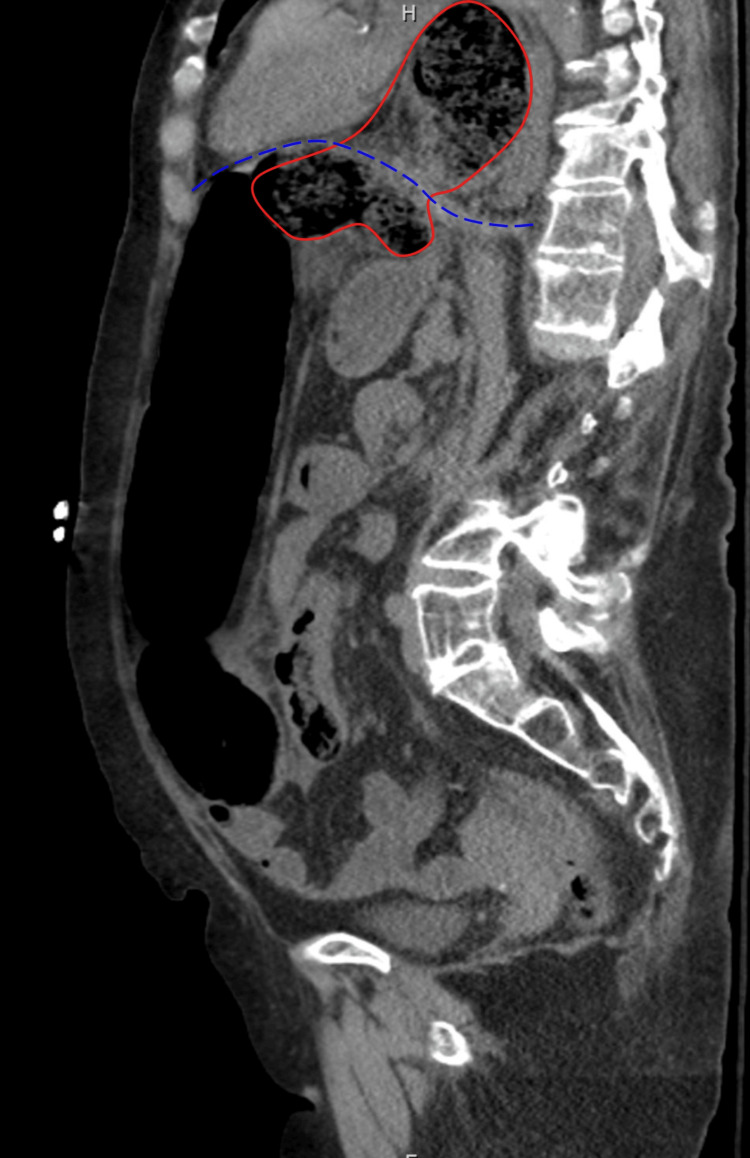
A sagittal view of the CT abdomen and pelvis shows a dilated bowel anteriorly (left) and a necrotizing bowel herniating into the thoracic cavity. The incarcerated apex of the sigmoid volvulus is shown, delineated in red. The diaphragm level is delineated by the dashed blue line. CT: computed tomography
